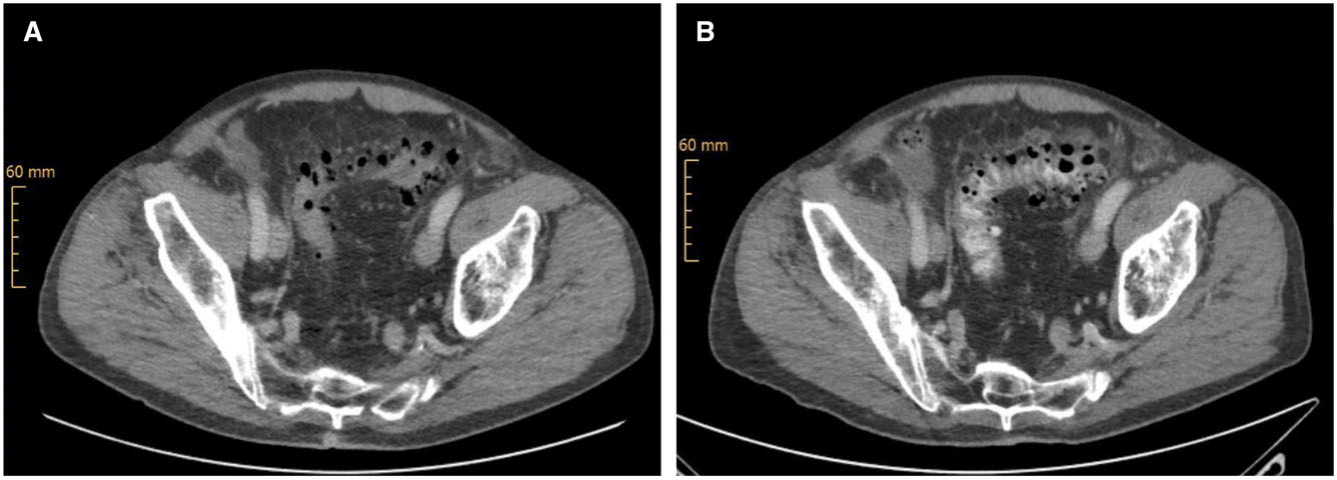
Computed tomography of the abdomen demonstrated wall thickening of the sigmoid and progressive pericolic fat stranding during hospitalization. (A) Imaging features at first presentation. (B) Imaging changes after 10 days of intravenous empiric antibiotic therapy.
